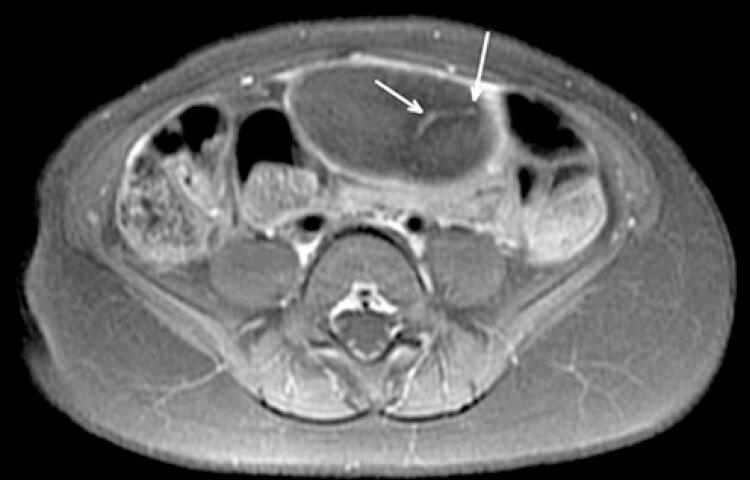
Magnetic resonance imaging, axial T1-weighted section, post-contrast with septum within the lesion and mild peripheral enhancement
